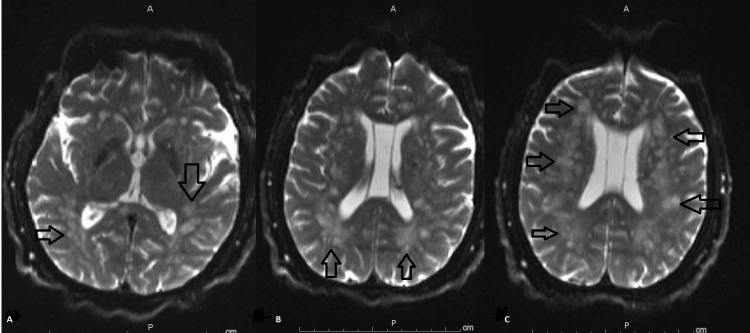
Further delineation of ischemic strokes on diffusion trace MRI brain Further delineation of the extent of punctate ischemic strokes on diffusion trace MRI brain involving cerebral hemispheres (arrows in A, B, and C)
